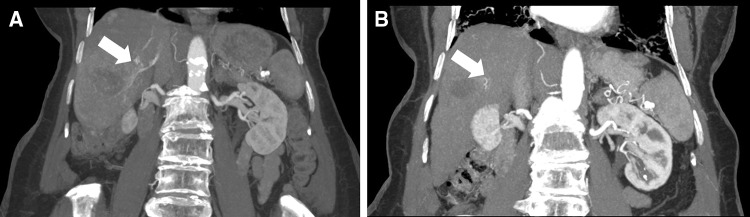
Coronary reconstructed maximum intensity projection of pre- and post-therapeutic CT of a 79-year-old patient with central HCC manifestations. A An arteriovenous fistula can be delineated based on the CT (white arrow). B After the treatment with sorafenib, the fistula cannot be visualized (white arrow)
